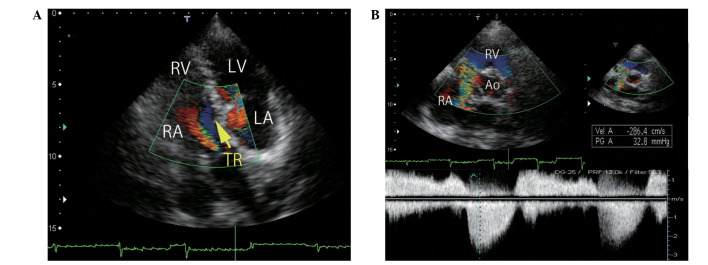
Echocardiographic imaging. (A) Color Doppler imaging of the four-chamber view reveals severe tricuspid regurgitation. (B) Continuous Wave Doppler revealing dagger-shaped curves with an early systolic peak velocity (2.9 m/s) and a rapid decline. RA, right atrium; RV, right ventricle; LA, left atrium; LV, left ventricle; TR, tricuspid regurgitation.
